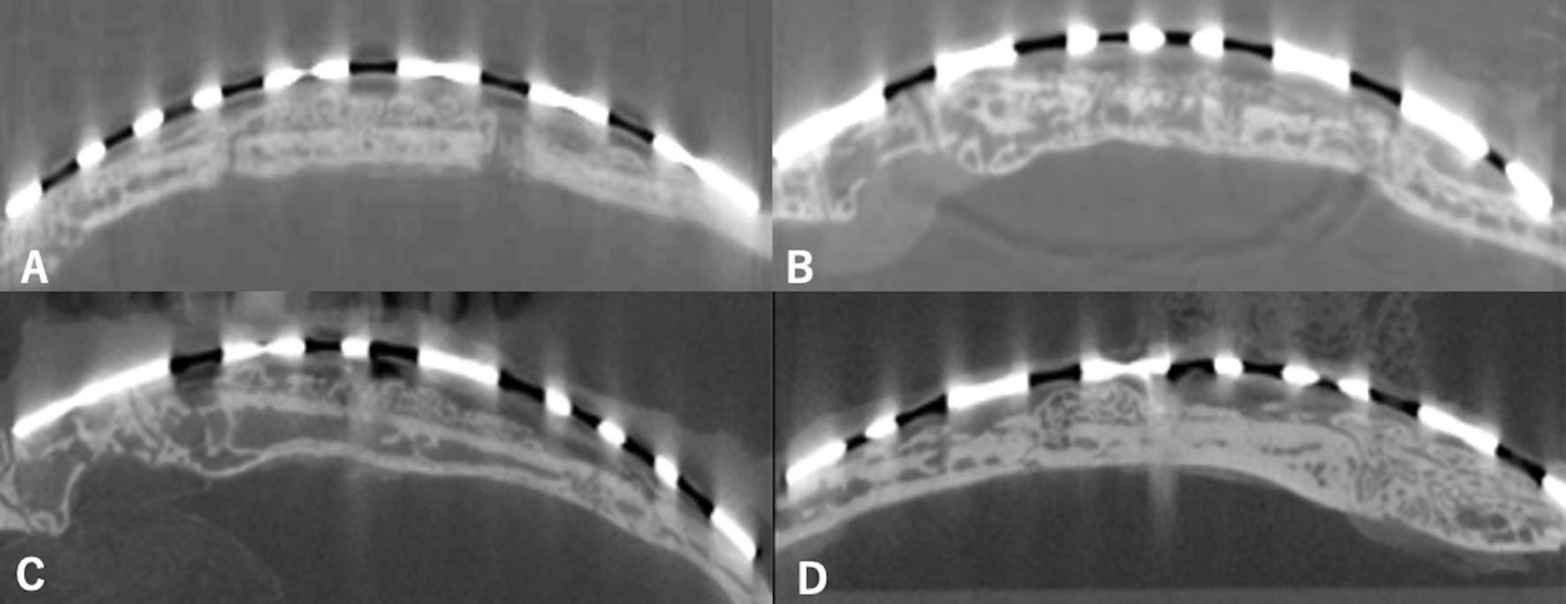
X-ray CT images showing a sagittal cross-sectional view of a dome-shaped bone with the same curvature as the original mesh at 5 weeks postoperatively in C1 (A), 8 weeks postoperatively in C2 (B), 5 weeks postoperatively in E1 (C), and 8 weeks postoperatively in E2 (D)
